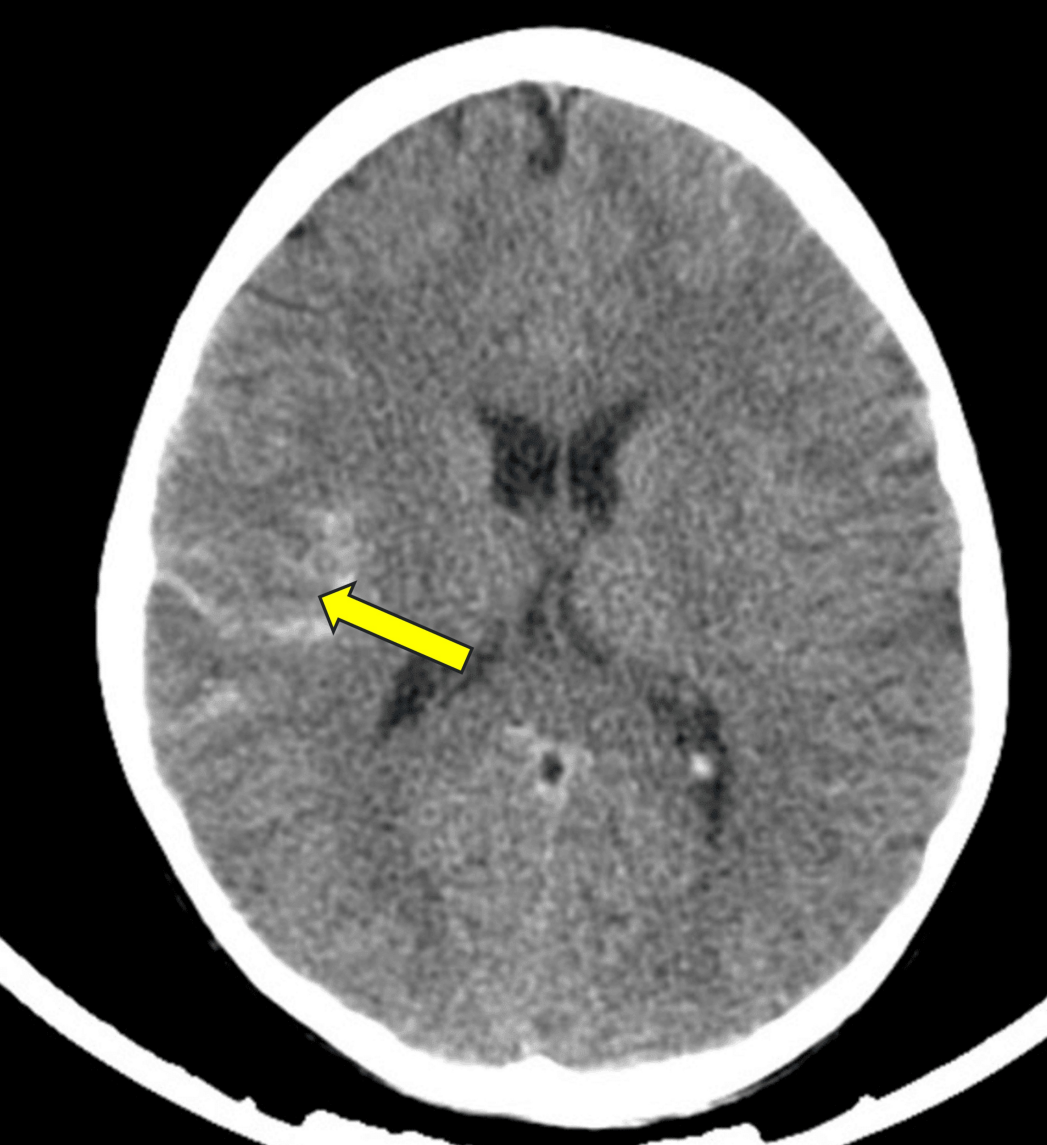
Non-contrast brain CT scan of case 1. The image demonstrates smearing of the right fronto-parietal and parietal cortical sulci by hyperdense material, indicative of subarachnoid hemorrhage.
